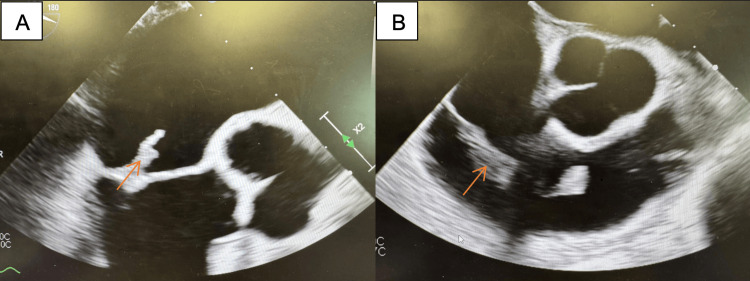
Transesophageal echocardiogram images (A) Mitral valve vegetation (1.6-1.7 cm); (B) tricuspid valve vegetation (4.5 cm).
